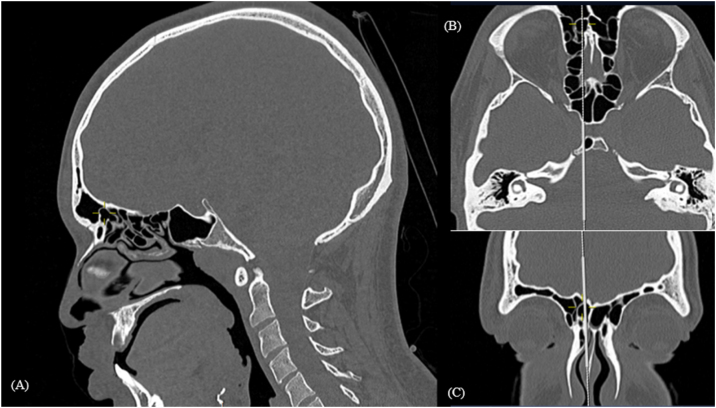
Supra agger frontal cell. Computed tomography: mark shows right supra agger frontal cell in sagittal (A), axial (B) and coronal (C) reconstructions.
